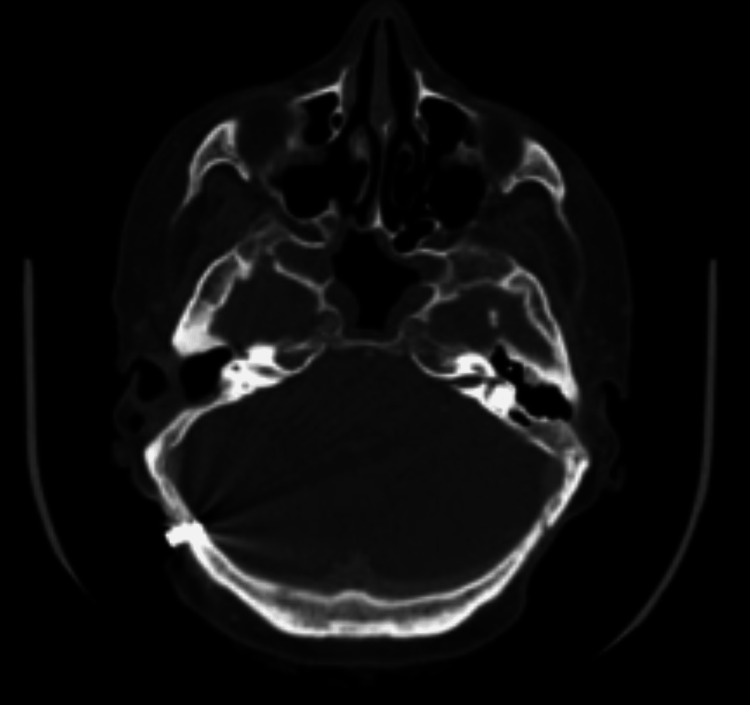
Right BAHA implant visualized on CT scan prior to bisphosphonate therapy on June 23, 2010 BAHA: Bone-anchored hearing aids.
